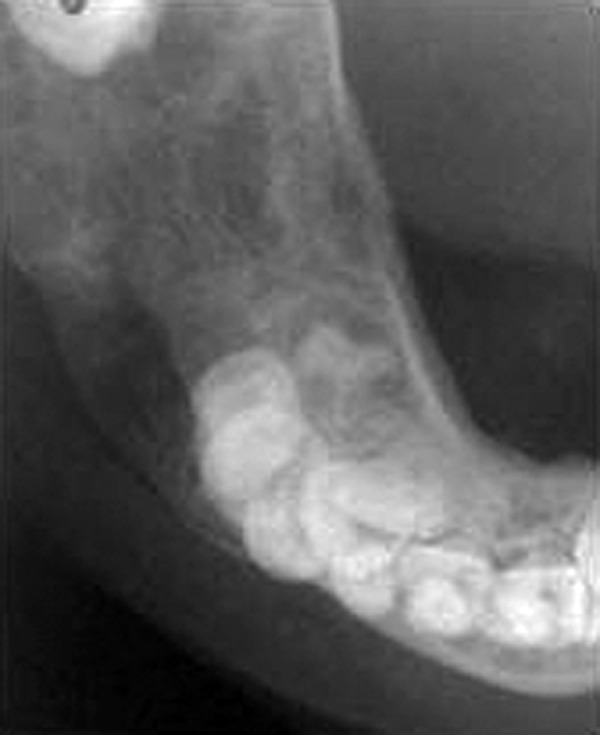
Cropped post-treatment occlusal radiograph shows intact cortex and mixed radiodensity in internal structure
